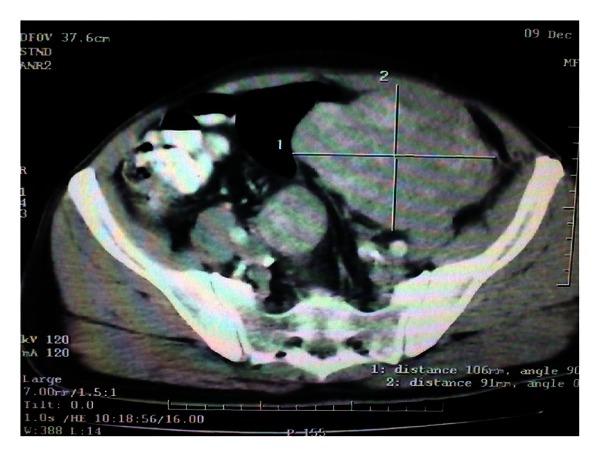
CT scan depicting the left and right iliac fossa mass of the patient.
